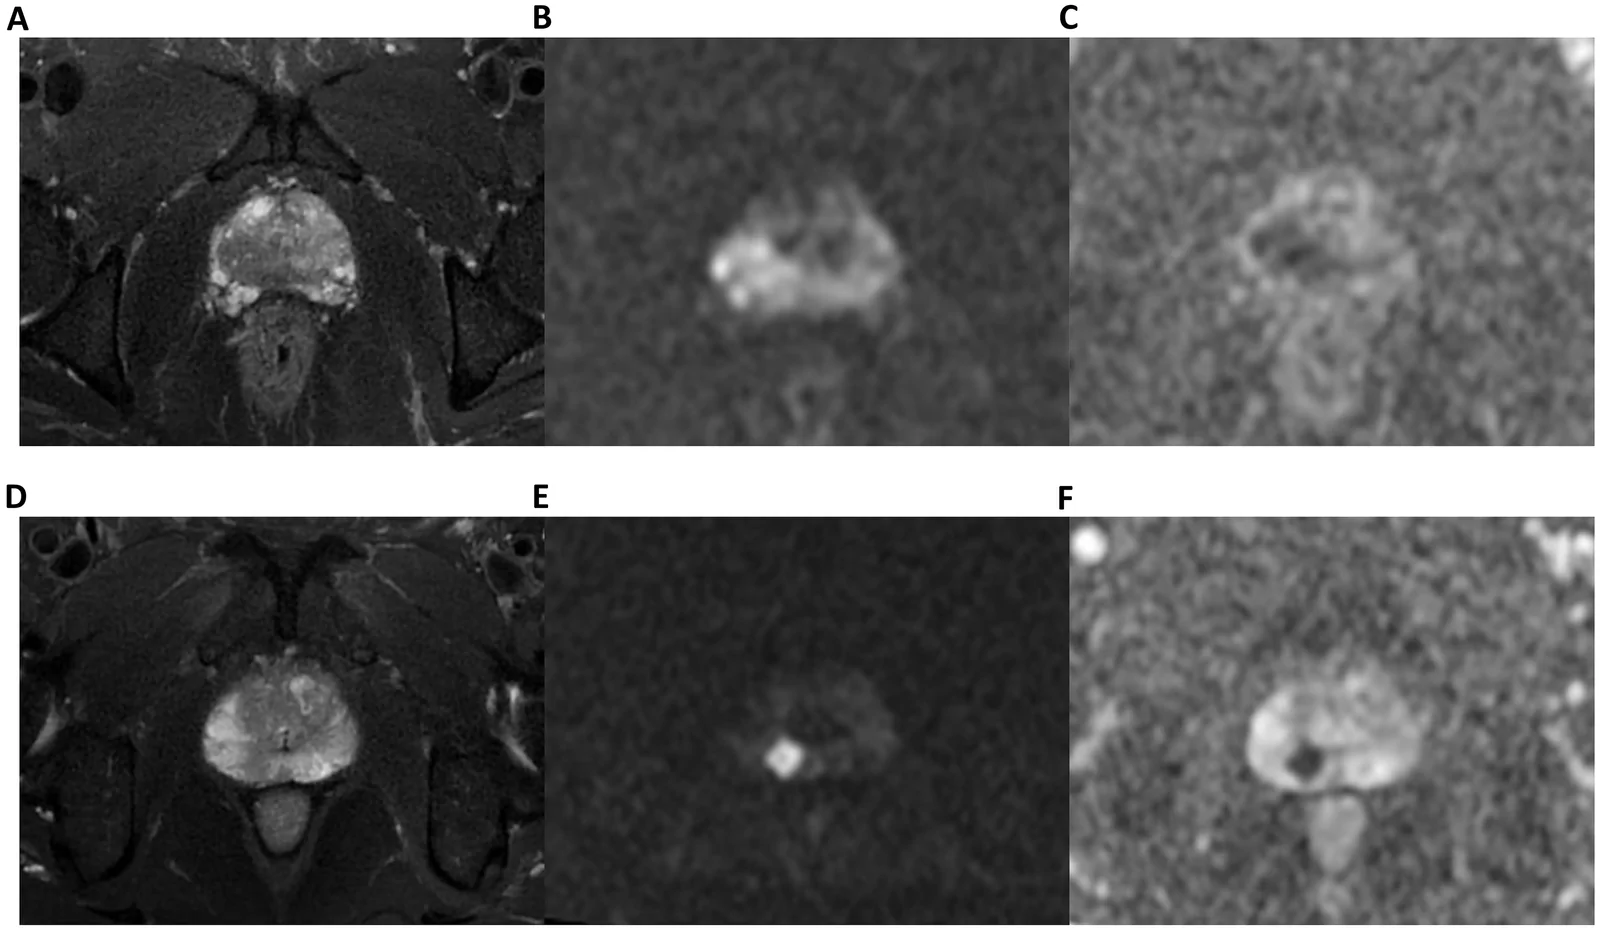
A 65-year-old male with EPE-positive prostate cancer.​ The right peripheral zone lesion exhibits low signal intensity on T2WI (A), high signal intensity on DWI (B), and corresponding low signal intensity on ADC map (C). The lesion was assigned a PI-RADS score of 5 and an MRI-EPE score of 2. A 76-year-old male with EPE-negative prostate cancer. The right peripheral zone lesion exhibits low signal intensity on T2WI (D), high signal intensity on DWI (E), and corresponding low signal intensity on ADC map (F). The lesion was assigned a PI-RADS score of 5 and an MRI-EPE score of 0.
